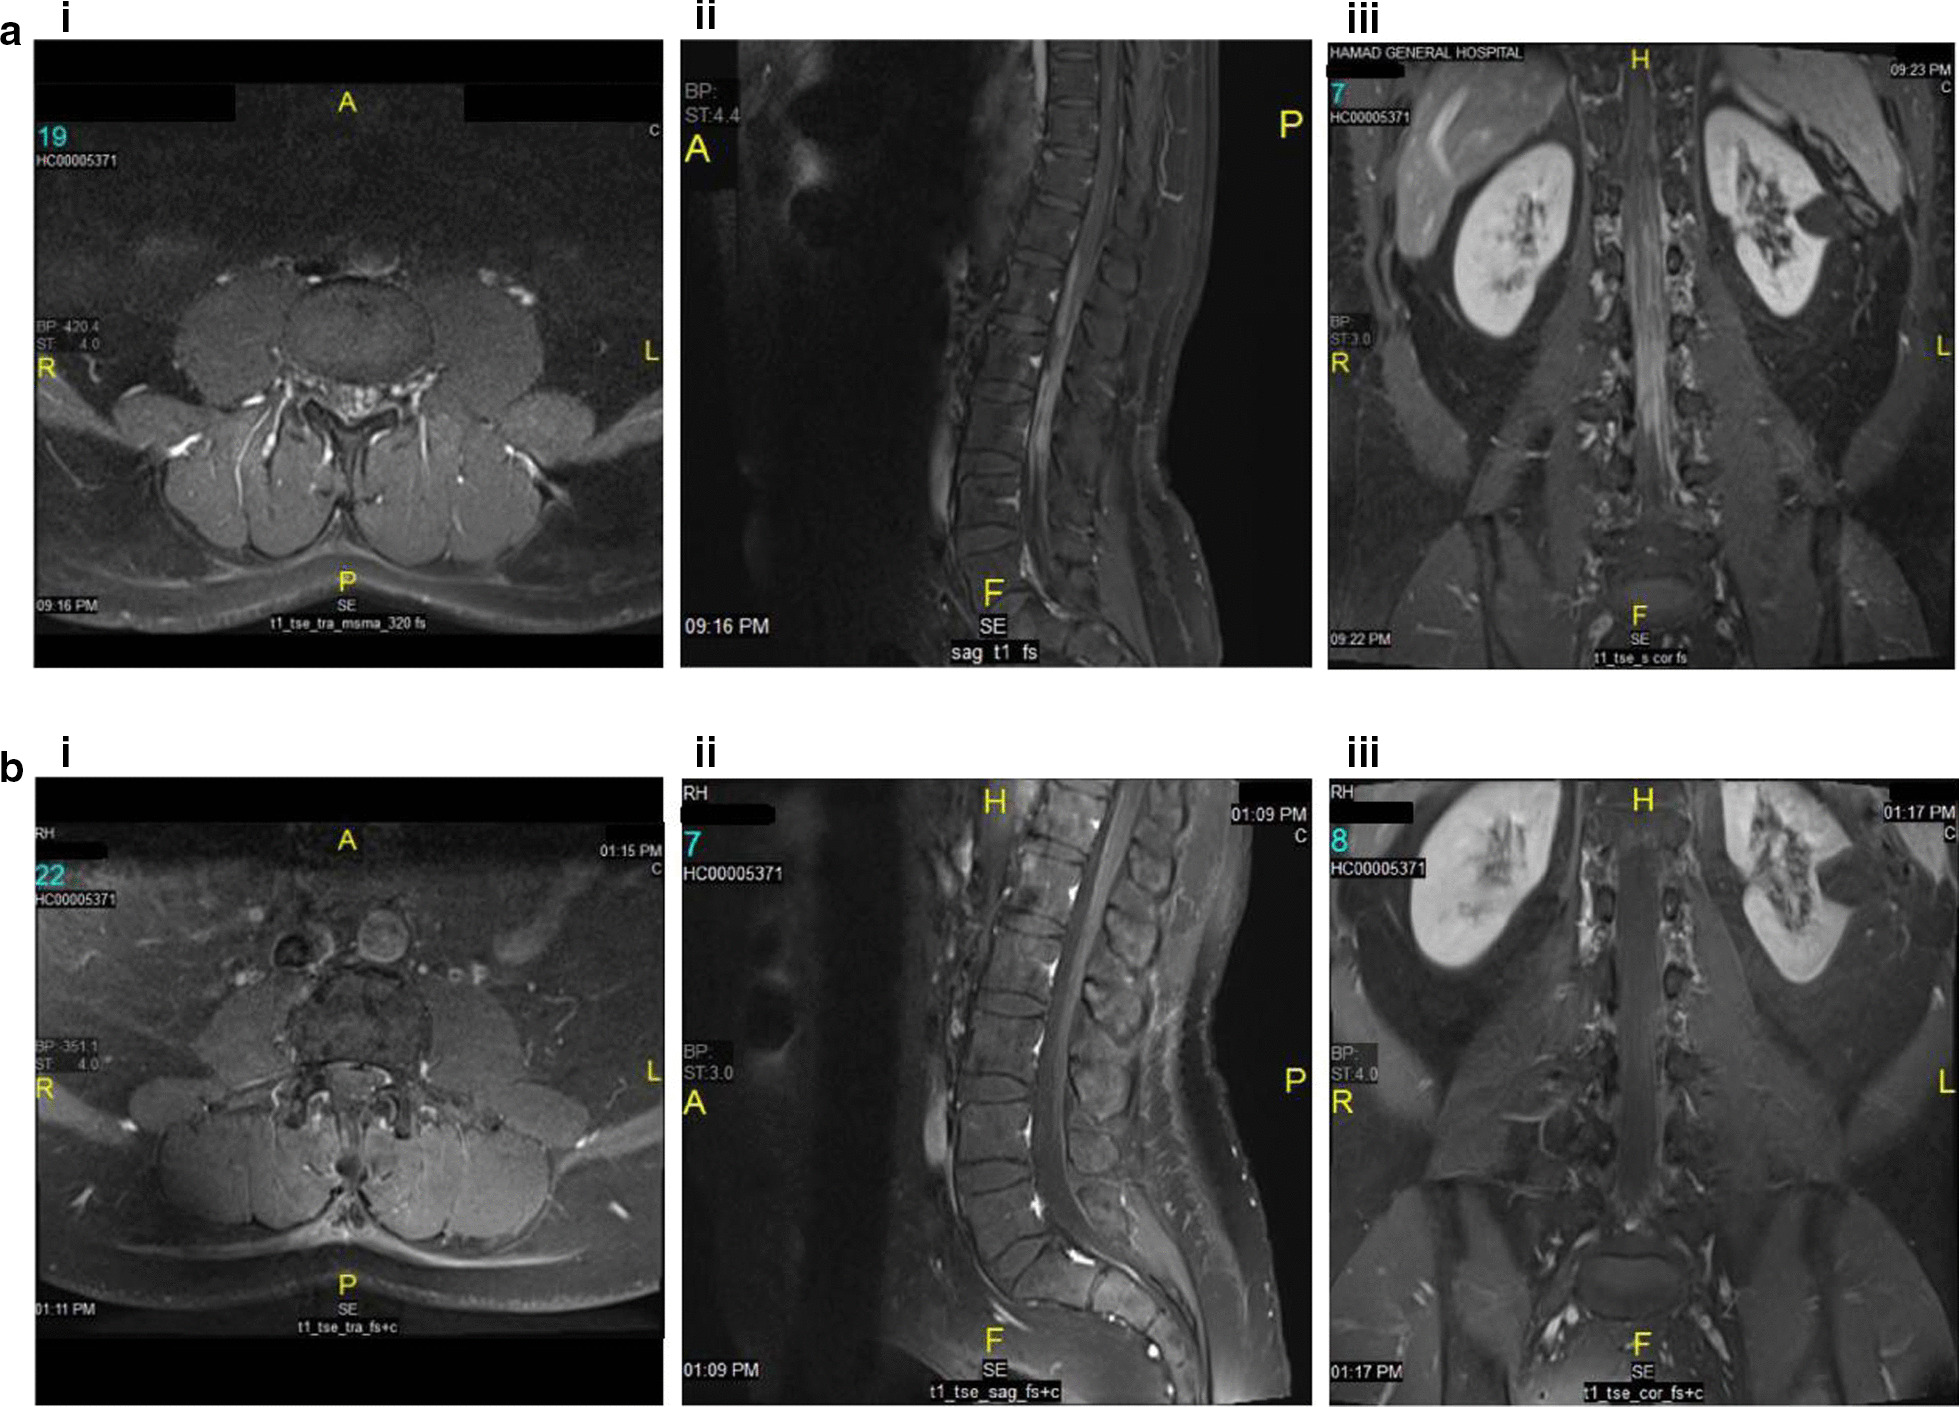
Case 3: radiological findings. a Post-contrast MRI studies show thickening and intense enhancement of the cauda equina nerve roots predominantly along the nerve sheath seen extending from the conus medullaris region down into the thecal sac to the lumbosacral plexus likely representing perineural tumor spread. Heterogeneous marrow signal pattern of the imaged vertebrae predominantly involving the lower cervical and upper thoracic vertebral bodies, showing bright signal intensity in T2- and STIR weighted images and heterogeneous mosaic pattern enhancement in the post-contrast series with no evidence of structural collapse or extra-osseous soft tissue component. b Post-contrast MRI study 33 months post-autologous stem cell transplant shows almost complete resolution of previously described residual thickening and enhancement of cauda equina nerve roots
